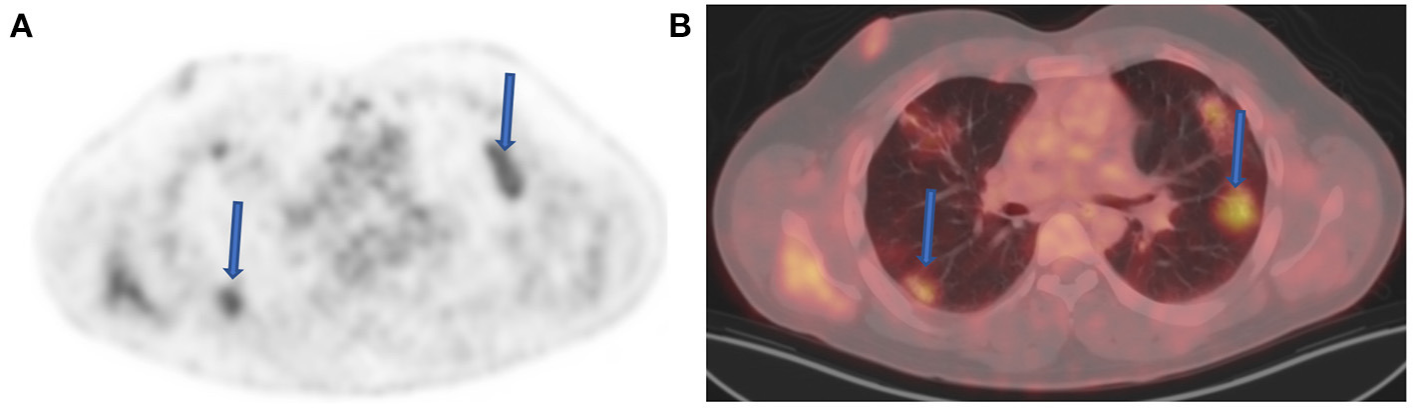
Ground-glass opacities (GGOs) with inflammation. FDG PET (A) and fused FDG PET-CT (B) images demonstrate increased FDG uptake within several bilateral foci of GGOs (arrows) in a 42-year-old male patient referred for post therapy evaluation. He indicated to have been diagnosed with coronavirus disease 2019 (COVID-19) infection 2 months before the study. Despite changes seen above, the patient was asymptomatic and did not have a lingering active chest infection.
